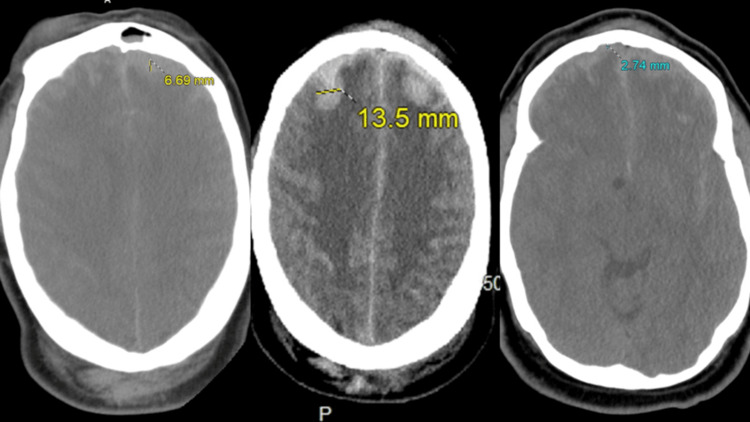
Axial head CT Head CT showing thin bifrontal subdural hematoma and subarachnoid hemorrhage with focal areas of hyperdensities along the inferior frontal lobes concerning for hemorrhagic contusions.
